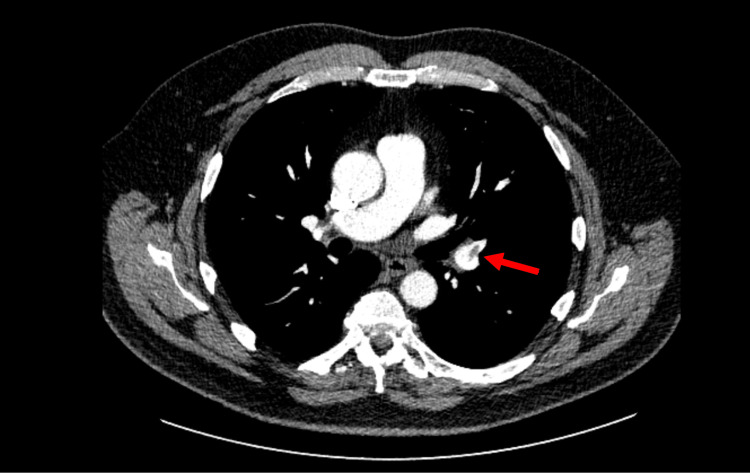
Computed tomography pulmonary angiography demonstrating pulmonary embolus. The red arrow marks filling defects in the left lobar artery suggestive of pulmonary embolism.
